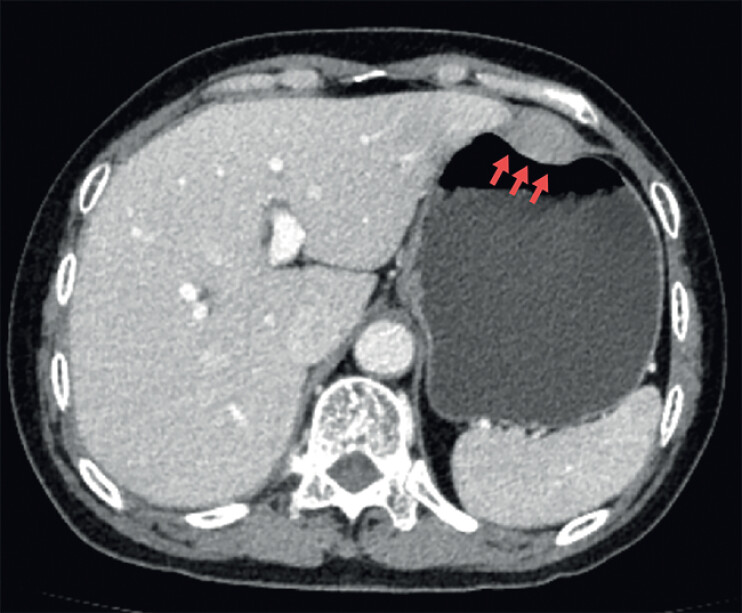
Preoperative computed tomography image showing a 3.5 × 2.5 cm extraluminal mass (arrow) along the greater curvature of the stomach.
